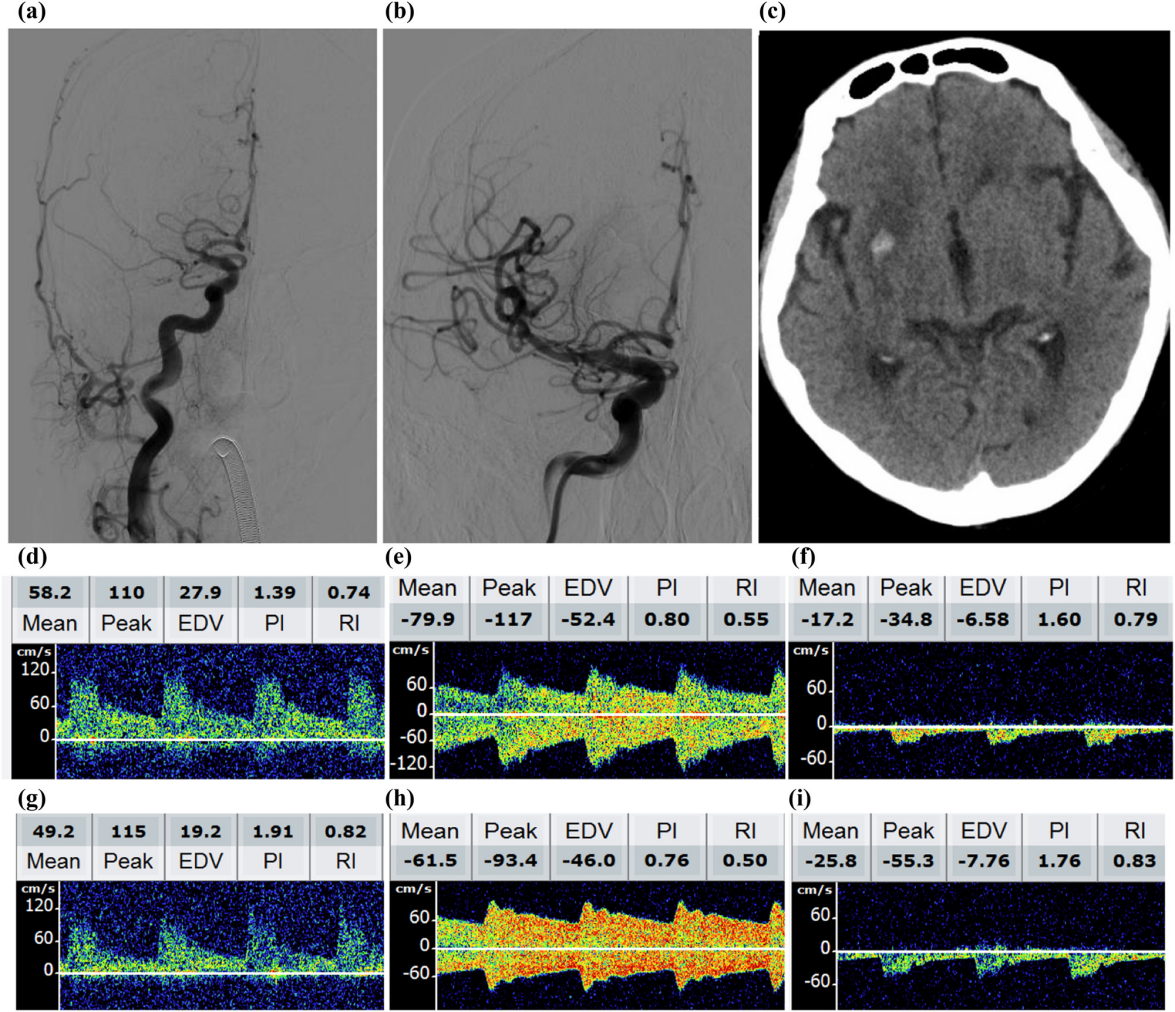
Representative images of a 78-year-old patient with MCA occlusion who underwent mechanical thrombectomy and achieved a 90-day mRS score of 0. (a) Pre-surgical DSA confirmed proximal MCA occlusion (right). (b) DSA after successful recanalization with a solitaire stent retriever shows MCA reperfusion. (c) CT at 24 h after mechanical thrombectomy shows nonsymptomatic ICH. Postinterventional transcranial duplex sonography 7 days after recanalization shows the ipsilateral (i) MCA (d), contralateral (c) MCA (g), a much higher c ACA SBF, and cACA RI (h) compared with iACA (e); iPCA SBF velocity index and much lower iPCA SBF (e) compared with the contralateral PCA (i).
